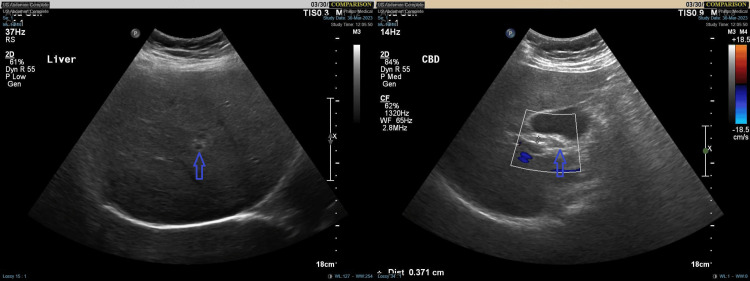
Abdominal ultrasound shows mild fatty liver, minimal gallbladder sludge without cholecystitis, normal common bile duct and portal vein, and no spleen abnormalities or free fluid.
